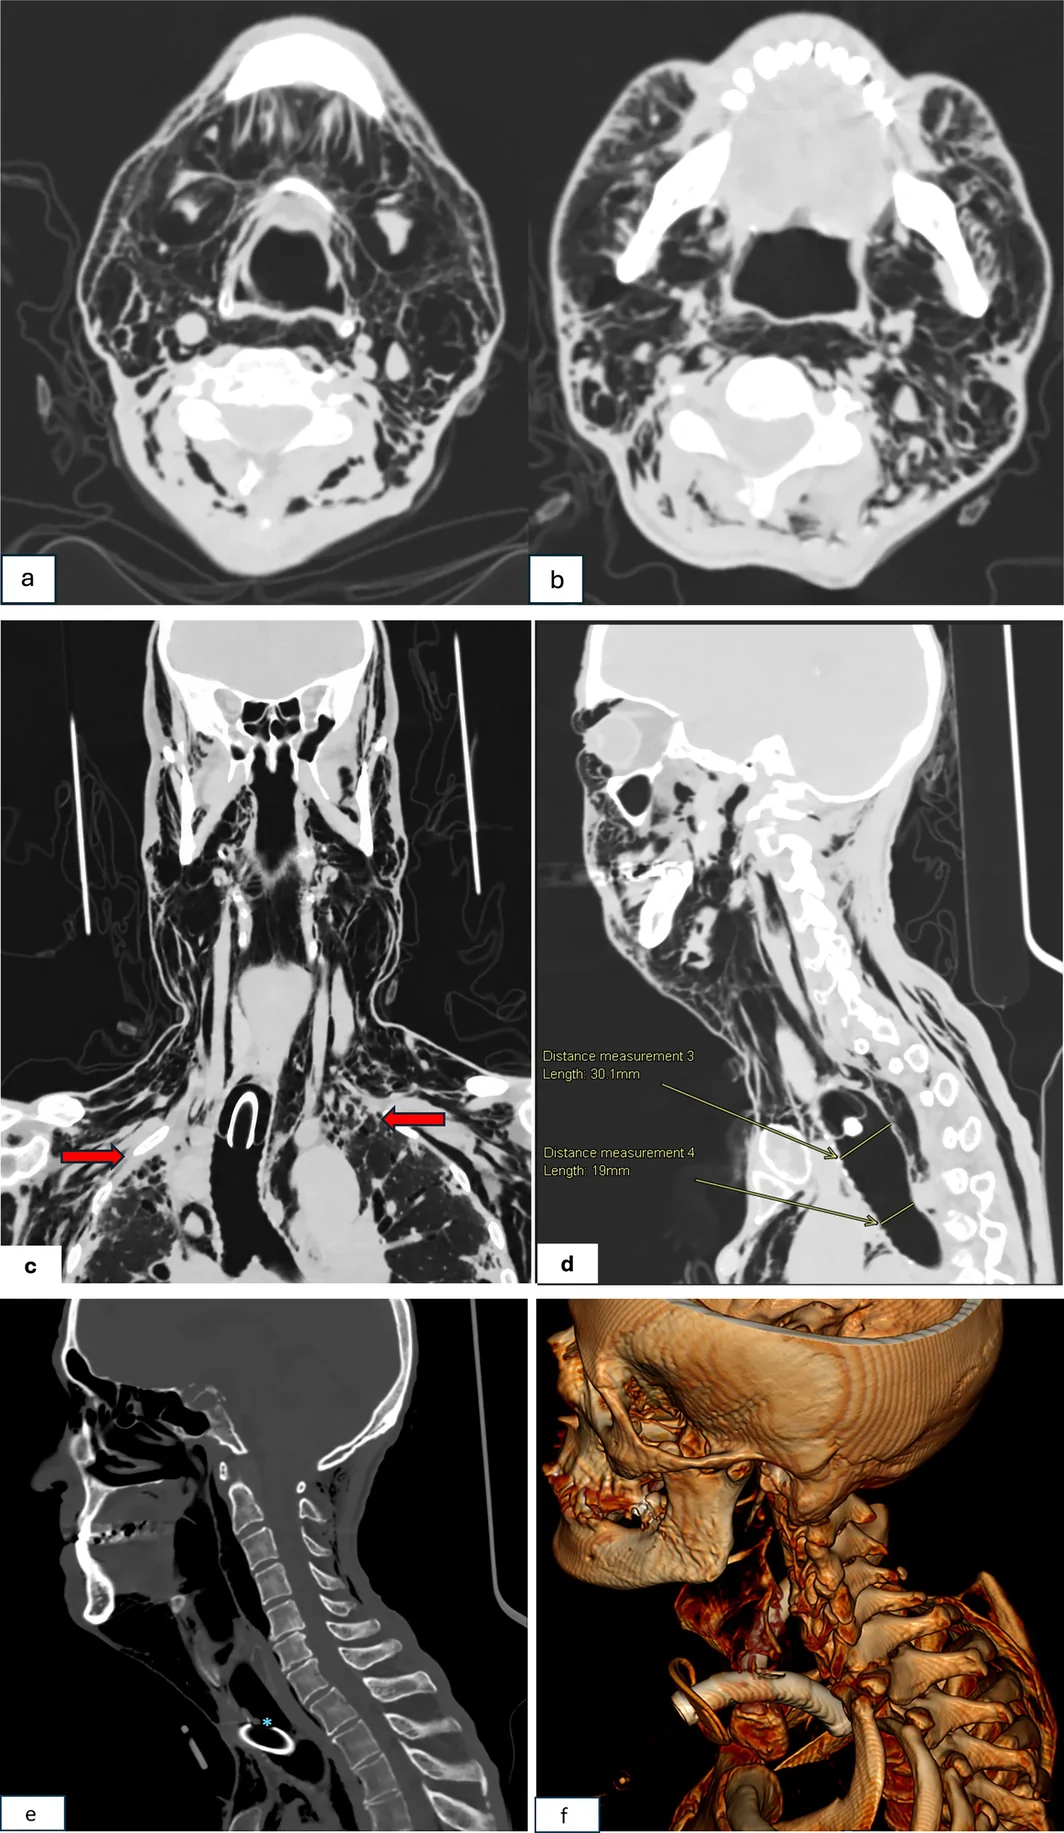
(a, b) Axial CT images of the neck at the oropharyngeal (a) and nasopharyngeal (b) levels demonstrate extensive subcutaneous emphysema with diffuse air dissecting throughout the superficial and deep neck spaces bilaterally. (c, d) Coronal CT of the neck and chest (c) demonstrates extensive cervicothoracic subcutaneous emphysema without evidence of pneumothorax at the lung apices (red arrows). Sagittal CT (d) demonstrating proximal tracheal widening with gradual reduction in caliber inferiorly. On this cut, the estimated anteroposterior tracheal diameter measures approximately 30 mm superiorly compared to approximately 19 mm inferiorly. (e, f) Sagittal CT images of the neck, bone window (e) and 3D reconstruction (f), show the upper fenestration of the tracheostomy positioned at the level of the anterior tracheal wall (blue asterix), with air extending into the surrounding soft tissues, suspicious for peri‐tubal air leakage. No focal tracheal wall defect.
